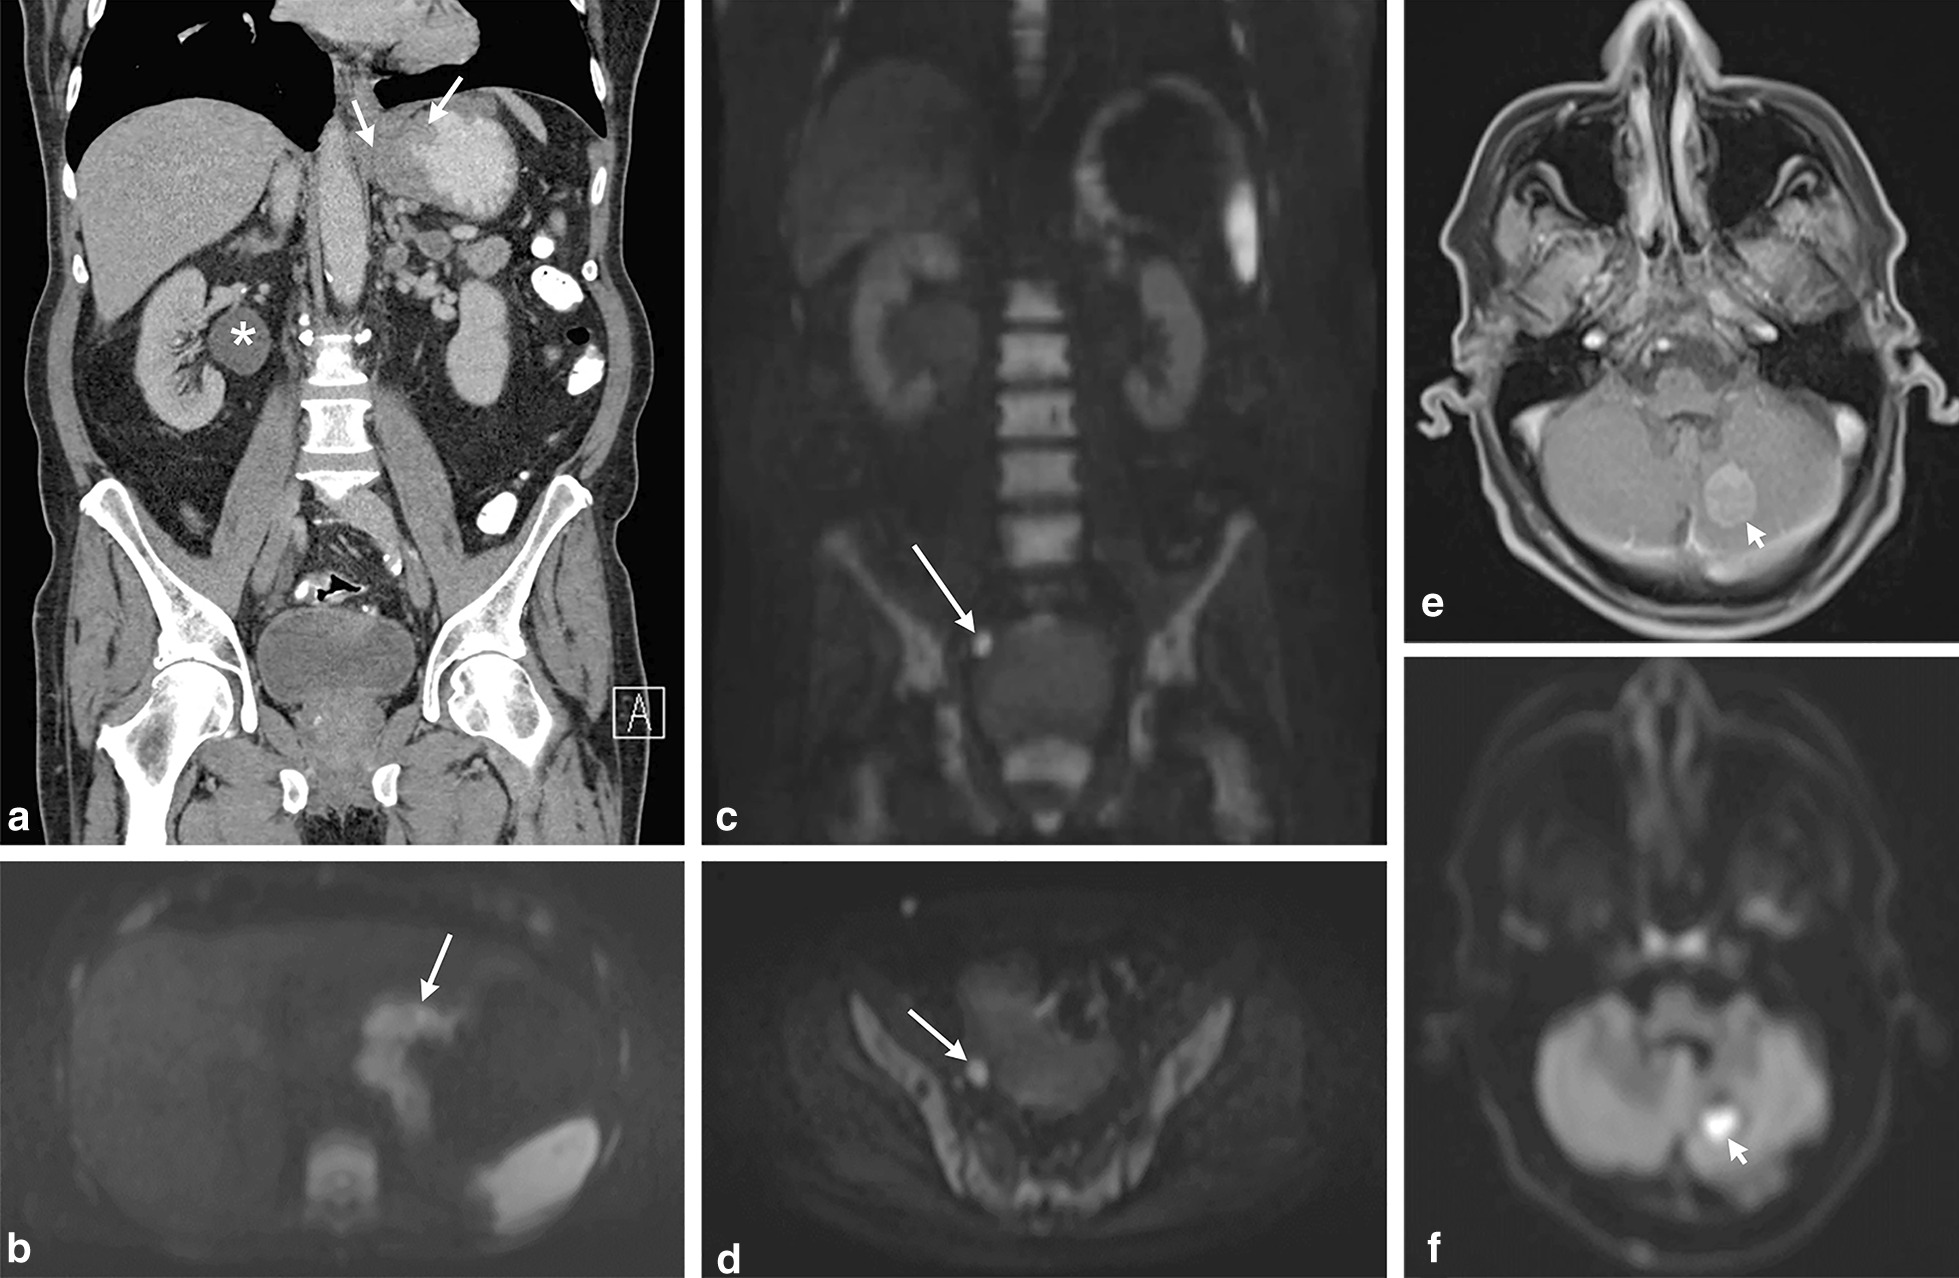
A patient with an age-range between 60 and 70 years old was diagnosed with a primary gastric adenocarcinoma with signet ring cell differentiation, localized at the cardia. CT scan (a) showed the primary tumor (arrows) as well as hydronephrosis of the right kidney (*), without a demonstrable cause. He had a WB-DWI/MRI for operability assessment. The primary gastric cancer is well visualized on the axial b1000 DWI (b, arrow). Apart from a peritoneal tumor implant on the right distal ureter (arrows) as the cause for the hydronephrosis on the coronal (c) and axial b1000 DWI (d), a brain metastasis (arrowheads) was found in the left cerebellum on the axial post-contrast T1 image (e) as well as the b1000 DWI (f), since the brain is included in the WB-DWI/MRI
